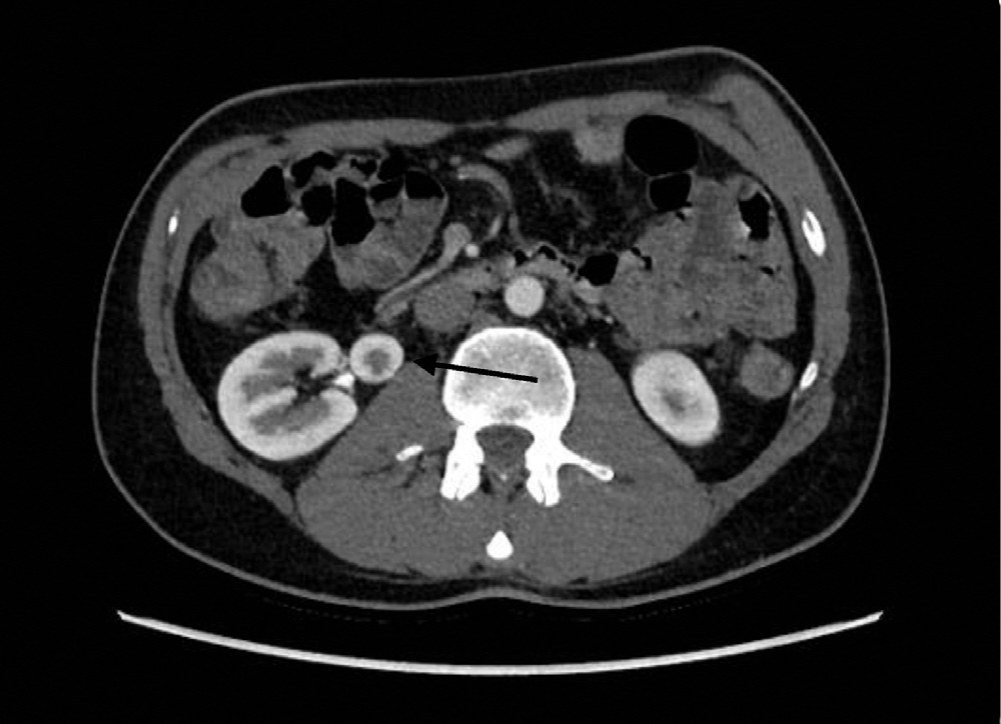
Axial image of computed tomography (CT) in post venous phase showing well defined lesion (arrow) medial to the right kidney. Post contrast enhancement compatible with adjacent kidney and similar morphology. The lesion demonstrates cortical and medullary differentiation as seen on the normal kidney.
